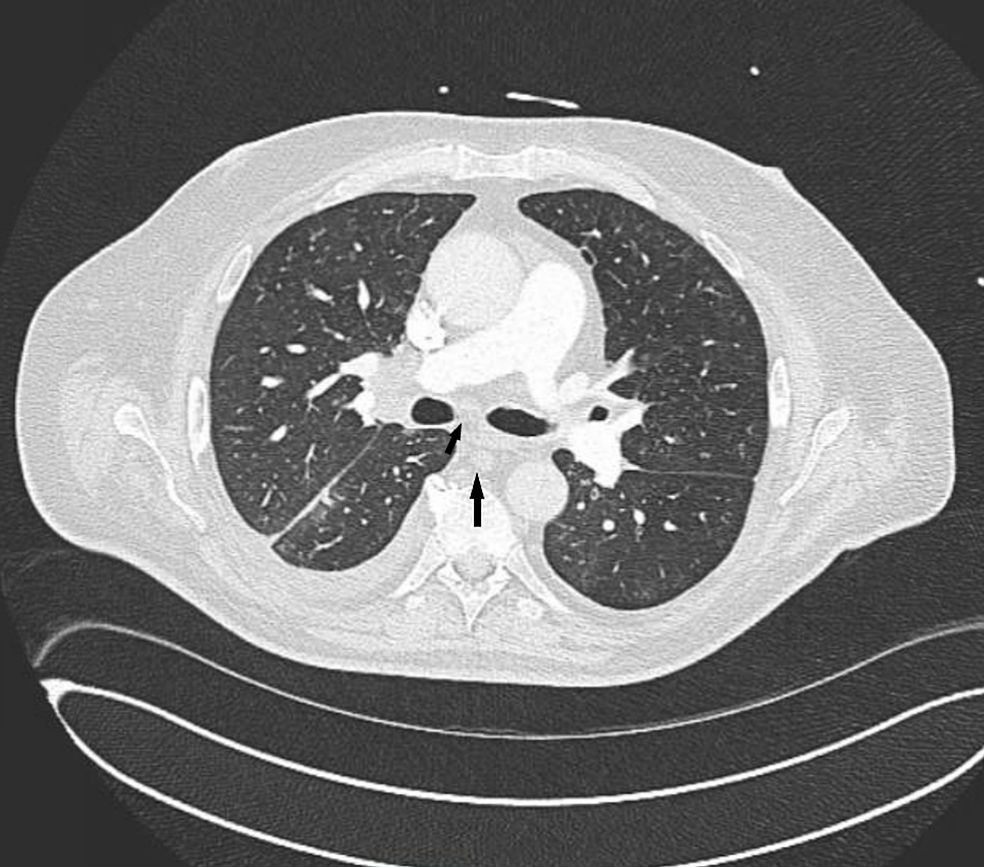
Axial view of CT-Angiogram on presentation, revealing hilar and infrahilar lymphadenopathy
